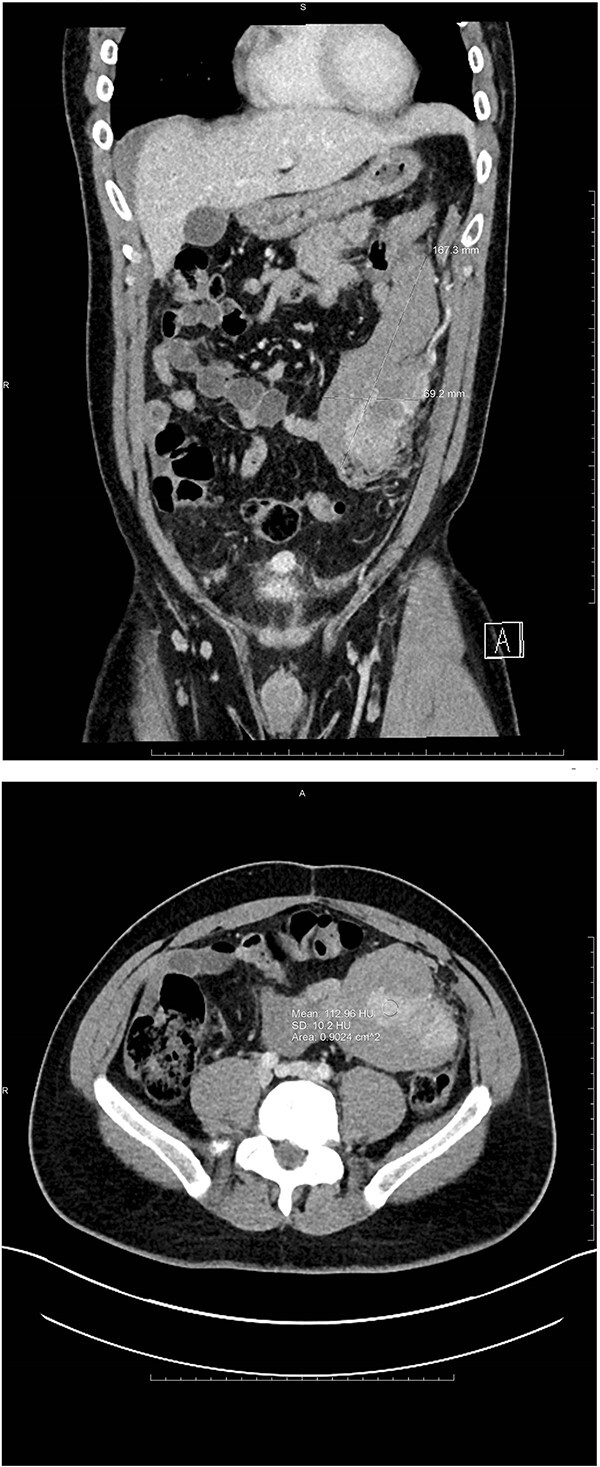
Coronal and axial views of CTA images of the abdomen and pelvis reveal a multilobulated heterogenous mass measuring 16.7 × 6.9 × 8.3 cm in the left mid-abdomen. Curvilinear foci of enhancement are visualized at its lateral aspect, originating from a prominent arterial vessel extending from the splenic artery.
